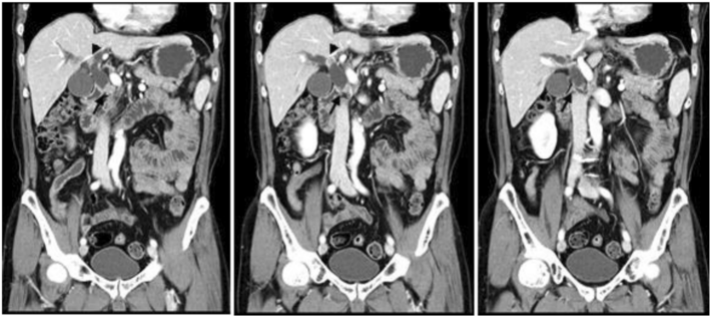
Abdominal computed tomography. Low density mass at common hepatic duct (black arrow head) and distal common bile duct (black arrow) with dilatation of intrahepatic duct and gall bladder.
